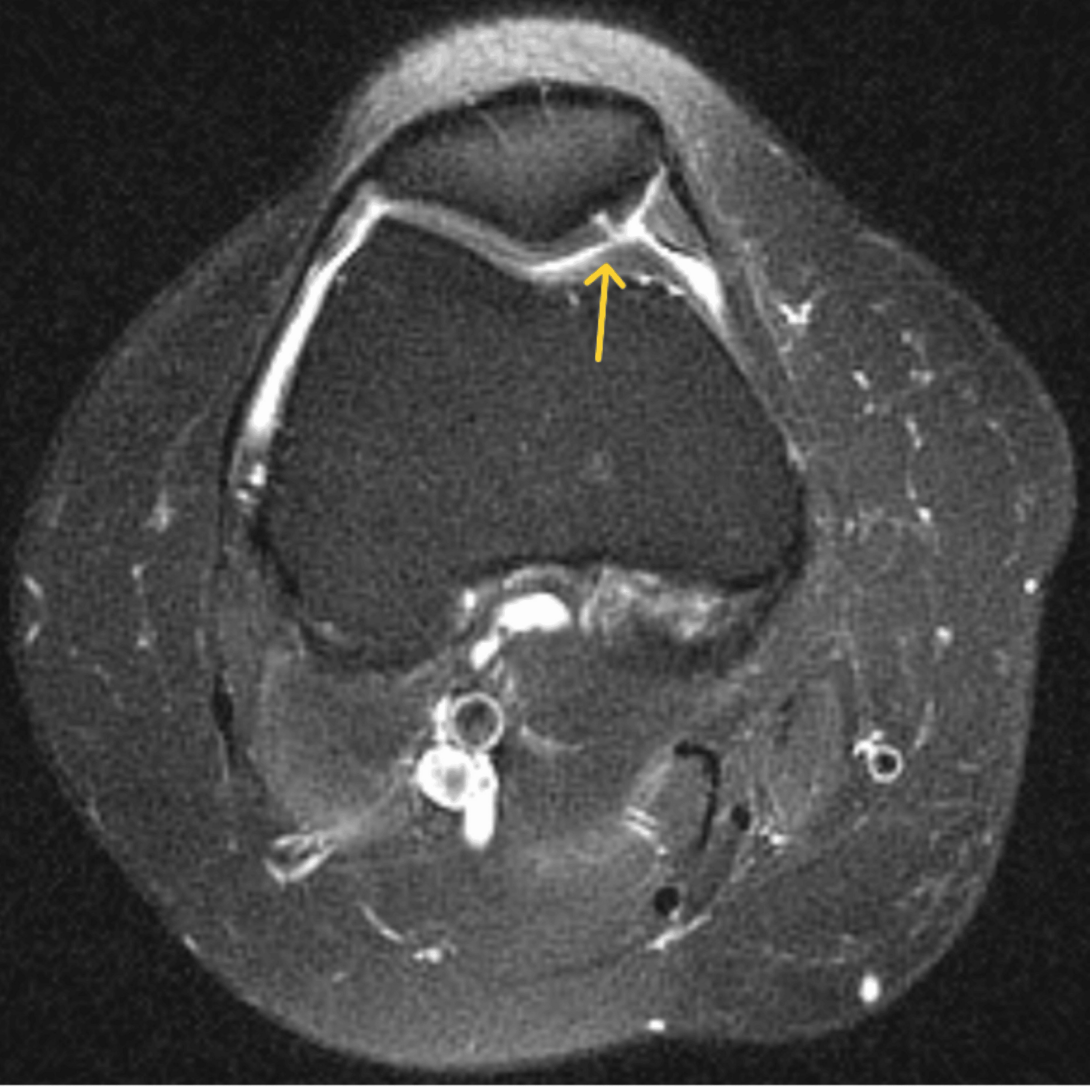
Axial T2-weighted MRI showing a full-thickness fissure in the medial patellar cartilage (yellow arrow)
